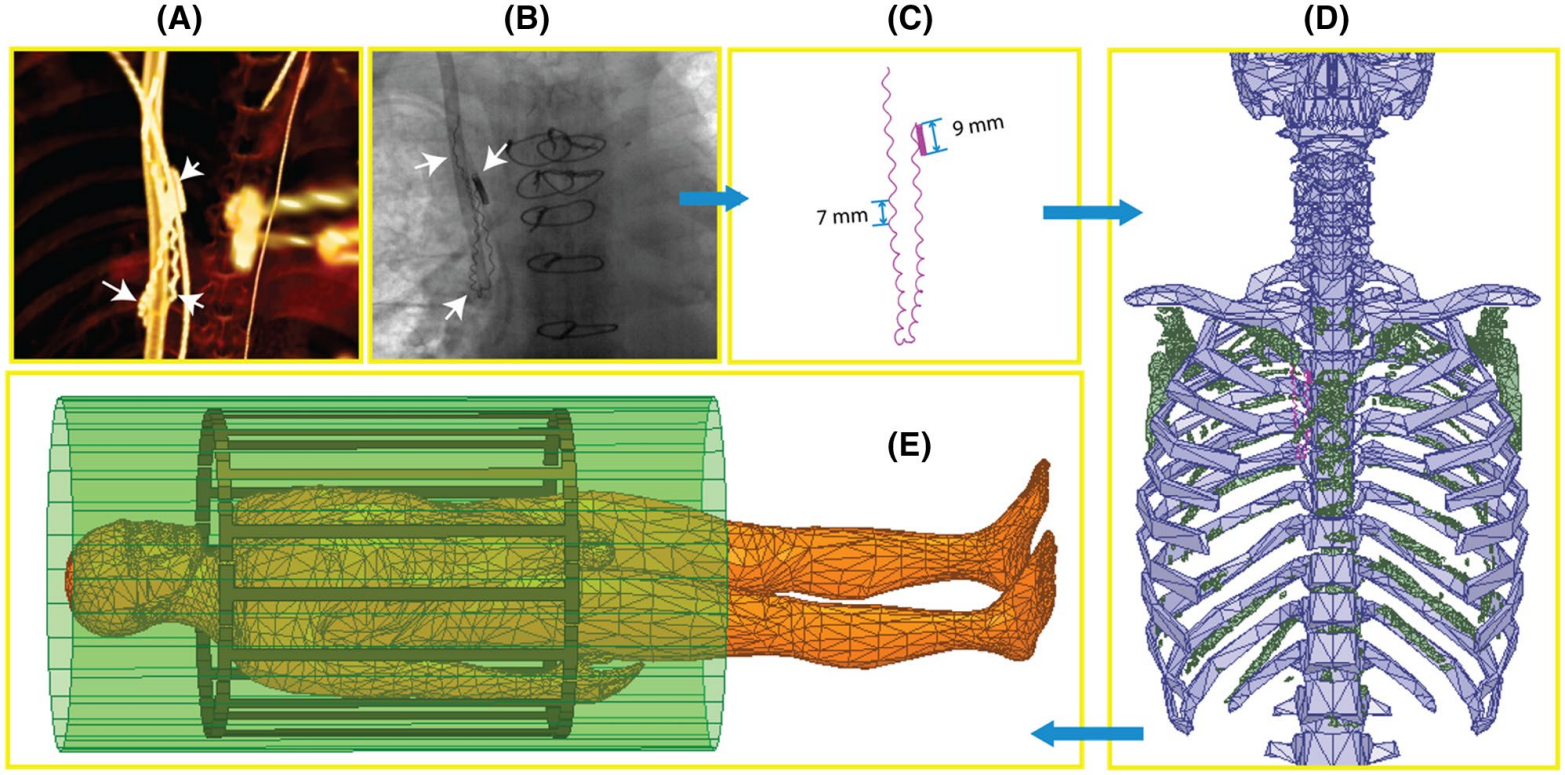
Steps of image segmentation and fragmented retained lead (FRL) model construction. (A–C) Computed tomography images were used to extract the 3D trajectory of FRL, whereas X‐ray images were used to reconstruct the FRL’s structure (e.g., number and pitch of loops). White arrows show the FRL on each image. (D) A triangulated surface of patient’s ribcage (green) was created and aligned with the ANSYS multicompartment body model (blue) to position the patient‐derived FRL model inside the ANSYS human body model. (E) Body model positioned inside the MRI coil
